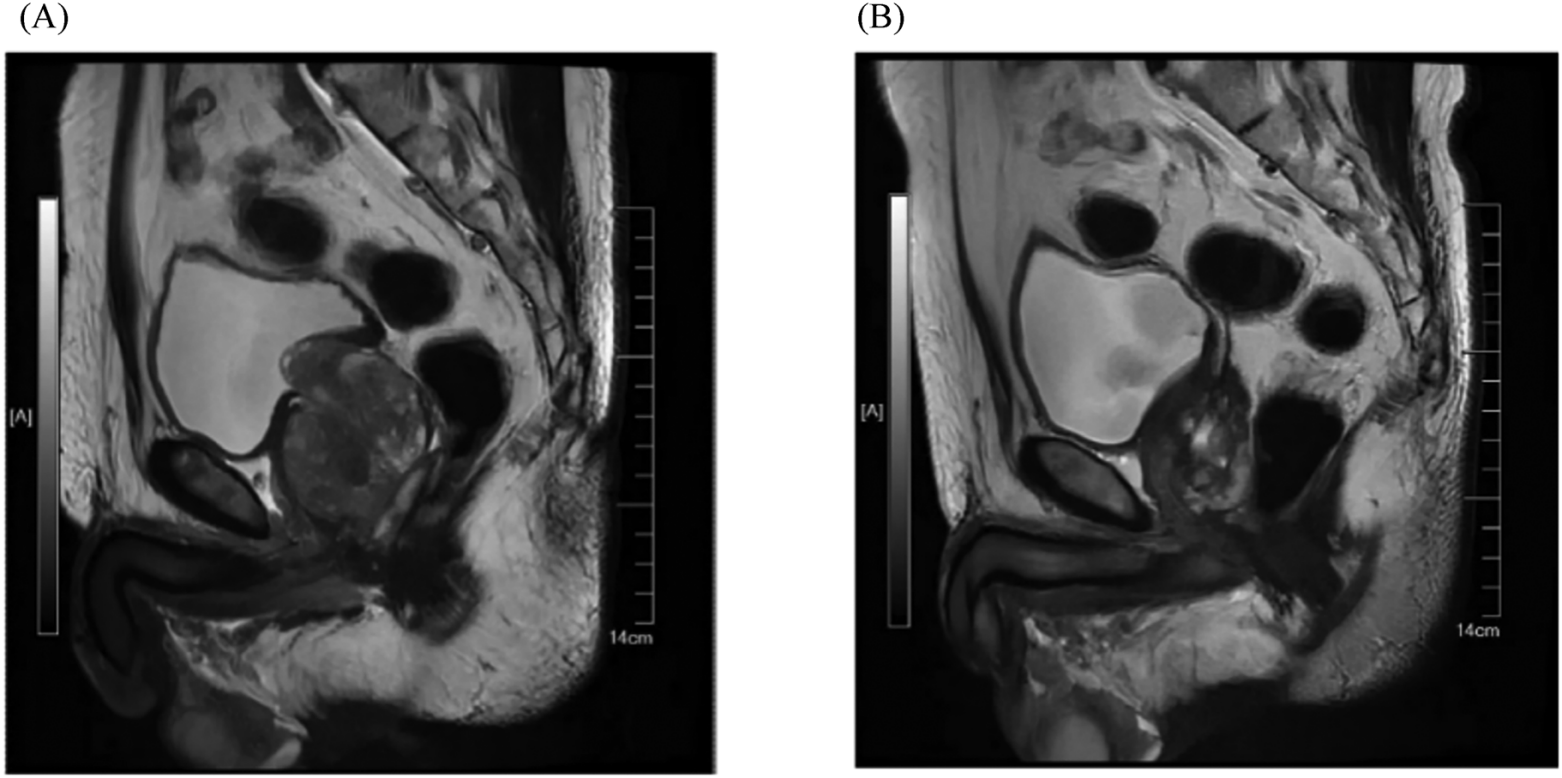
The magnetic resonance imaging (MRI) in sagittal plane of the prostate for the same patient before the operation (A) and 1 year after operation (B).
